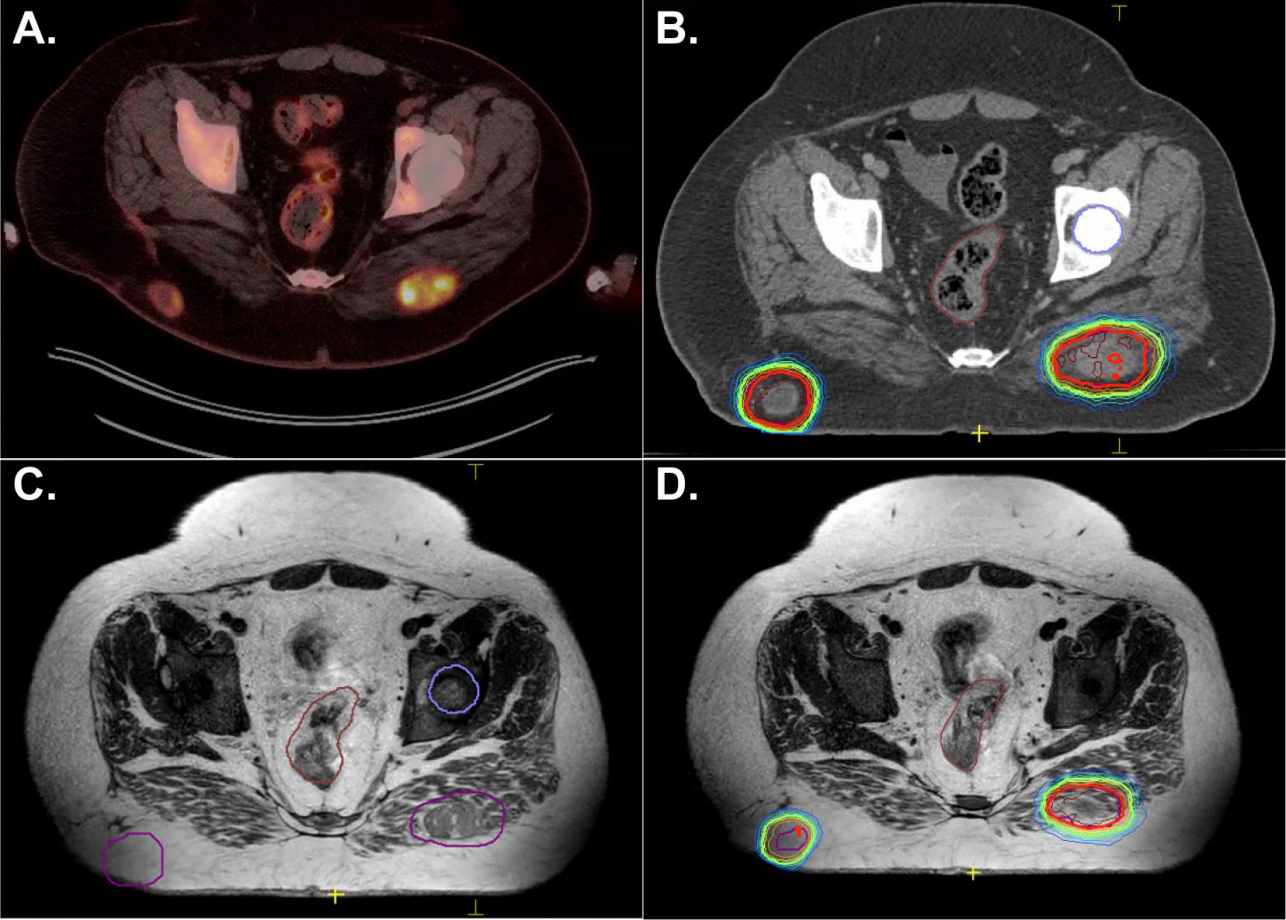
A 24-year-old male with metastatic Ewing sarcoma presented with two enlarging, painful gluteal metastases. Panel (A) shows the diagnostic PET scan. Panel (B) shows the lesions on CT. Notably, on both the PET scan and treatment planning scan, both nodules were visualized in the same plane. A single isocenter plan was created. Panel (C) shows the MRI obtained during the first fraction of treatment where the right-sided lesion is now out of the plane. Panel (D) shows successful adapt-to-shape for this single isocenter plan.
